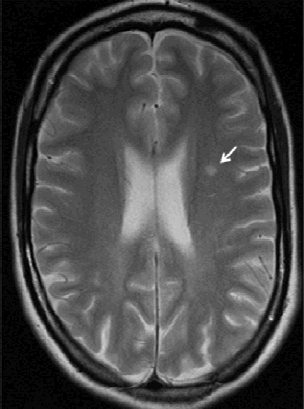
T2 weighed sagittal secion of brain MRI of patient I1 family 11: T2 hyperintense spot is present in the frontal white matter (arrow).
